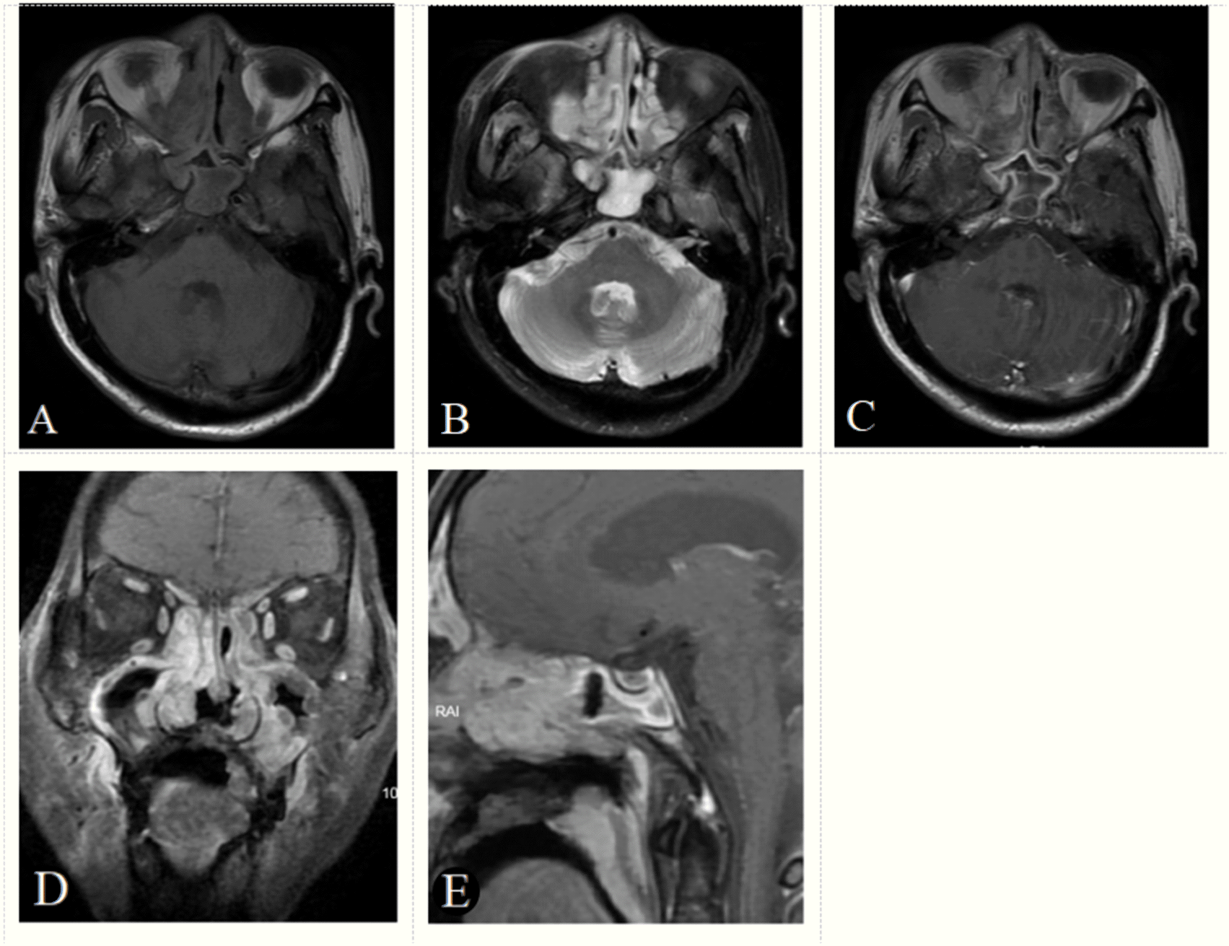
A 70-year-old male patient with positive EB virus nucleic acid and ENKTCL. The tumor appears as a diffuse growth of soft tissue masses in the bilateral nasal cavity and paranasal sinuses, with an isointense signal on axial T1-weighted image (A), a hyperintense signal (signal between muscle and nasal mucosa) on T2-weighted image (B), a heterogeneous enhancement on axial, coronal, sagittal enhancement T1-weighted image (C–E) and a gyrus like changes on sagittal enhancement T1-weighted image (E). The tumor boundaries are blurred and the tumor invades the maxilla and adjacent soft tissues. Based on the score model, this patient scored 16 points, the maximum in the points-scoring system.
